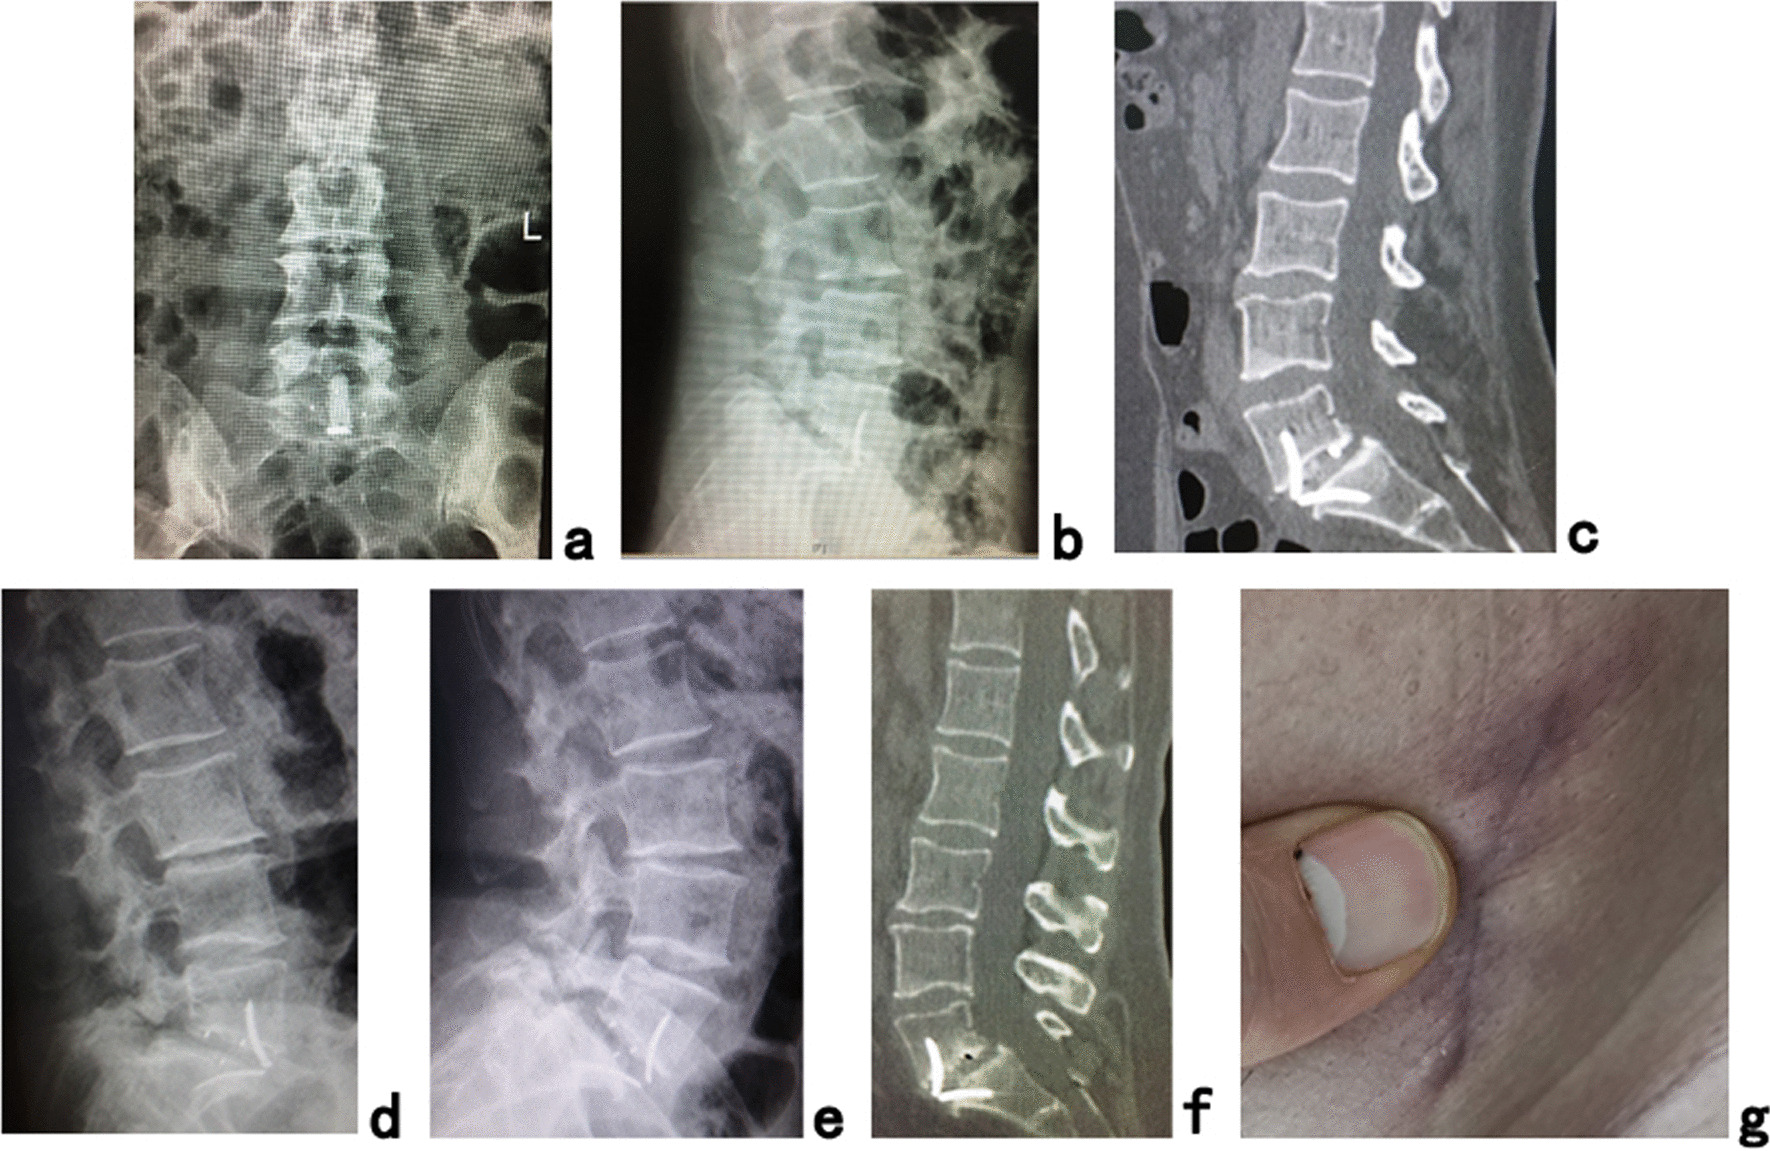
a Posteroanterior and b lateral X-ray images and c sagittal CT image showing good positioning of the cage immediately after the operation. No instability at the fusion level was found on d hyperflexion and e hyperextension lateral X-ray images. The fusion grade at the 2-year follow-up was grade I on the f sagittal CT image. g The picture shows the cosmetic incisions for OLIF51 (Case 3)
